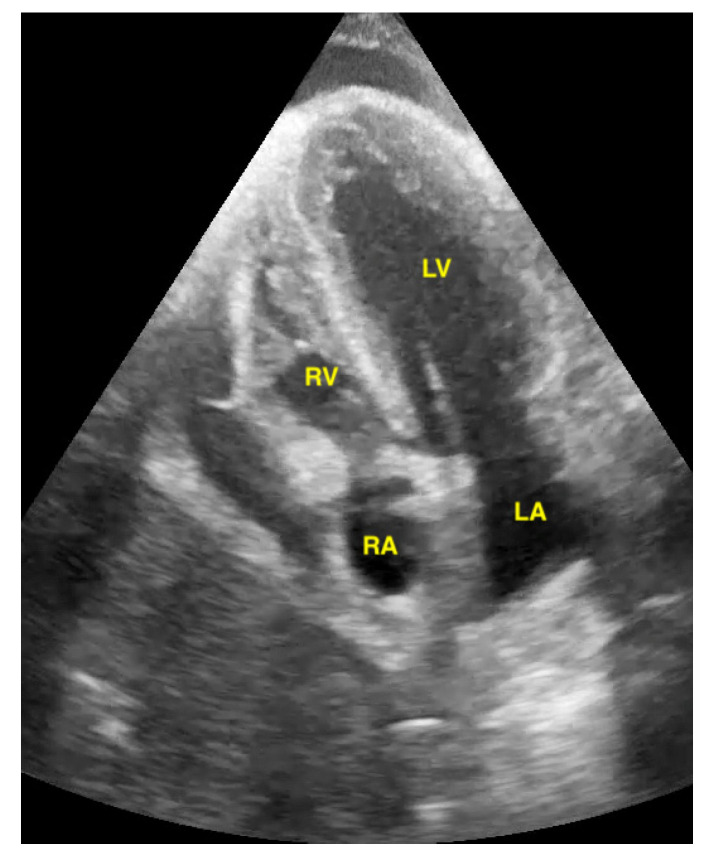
Pericardial effusion in apical four chamber view compresses the right atrium slightly (RA = right atrium, LA = left atrium, RV = right ventricle, LV = left ventricle).
